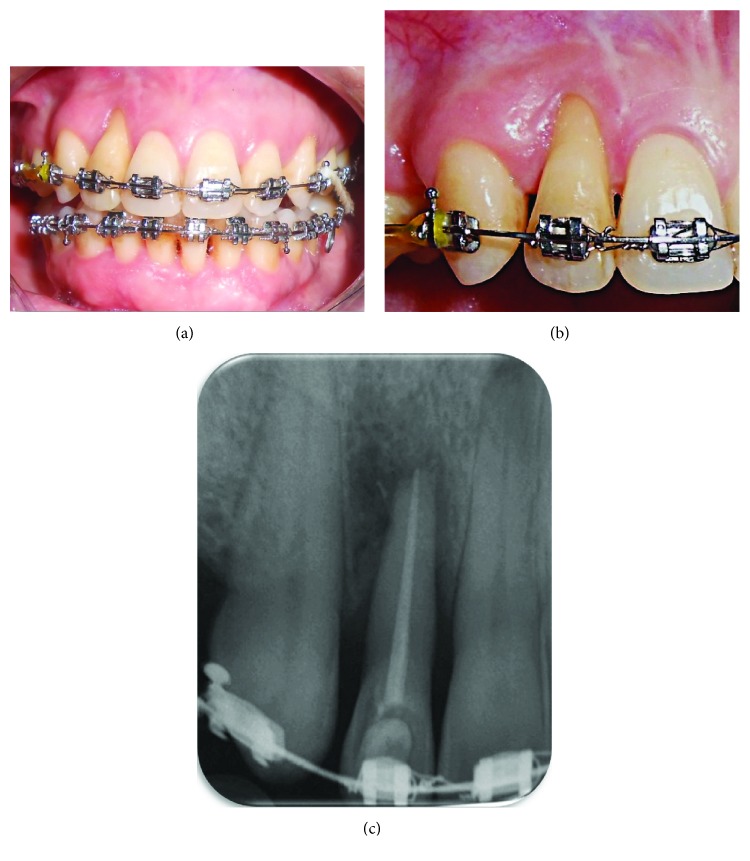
Two-year follow-up. (a, b) Facial view. Note a consistent recession reduction. (c) Periapical radiograph.
